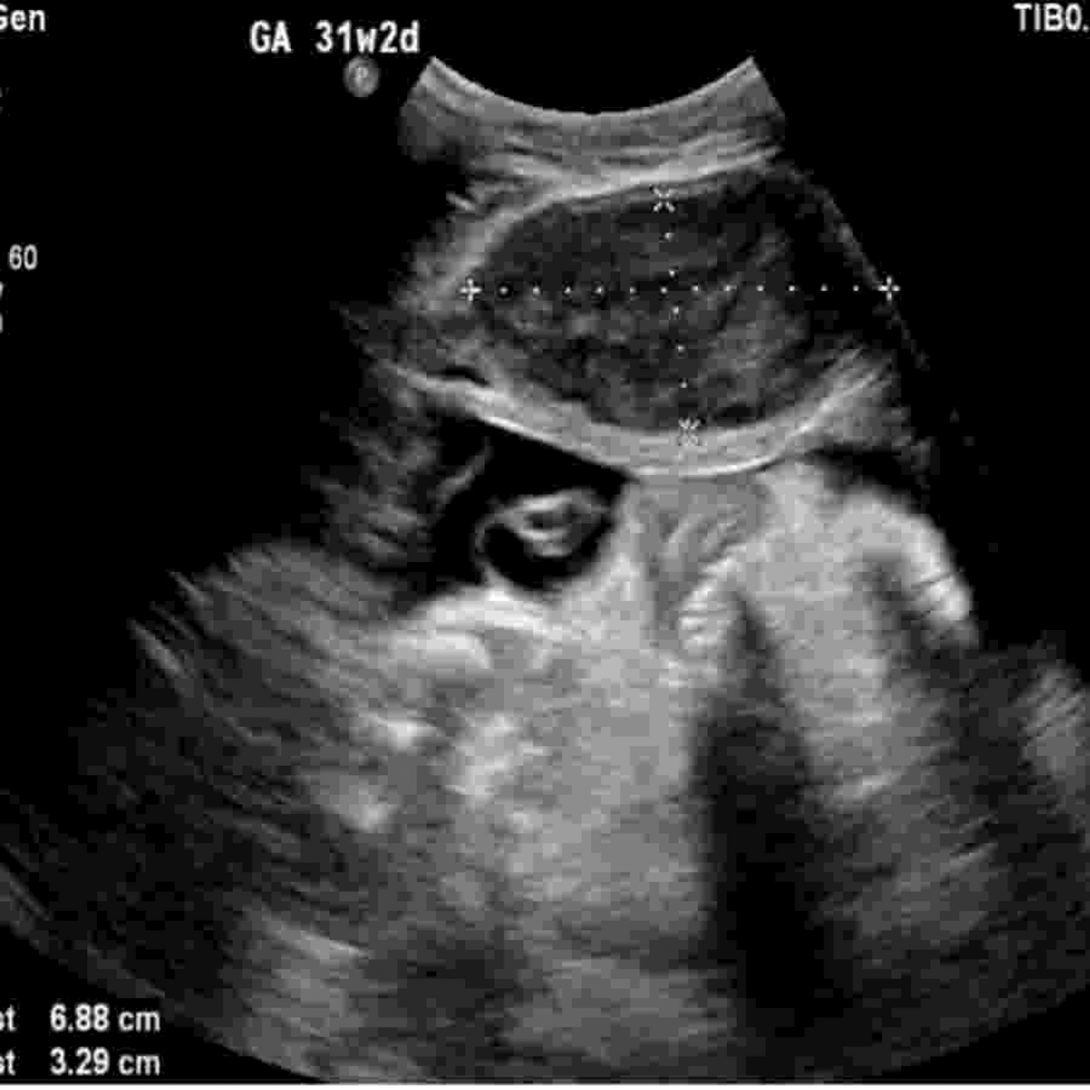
Ultrasound at 31 weeks and 2 days of gestation showing a second anterior uterine wall fibroid measuring approximately 6.88×3.29 cm. The fibroid appears as a well-defined hypoechoic mass, with no immediate compression effects on the fetus, suggesting a stable fibroid during this stage of pregnancy.
